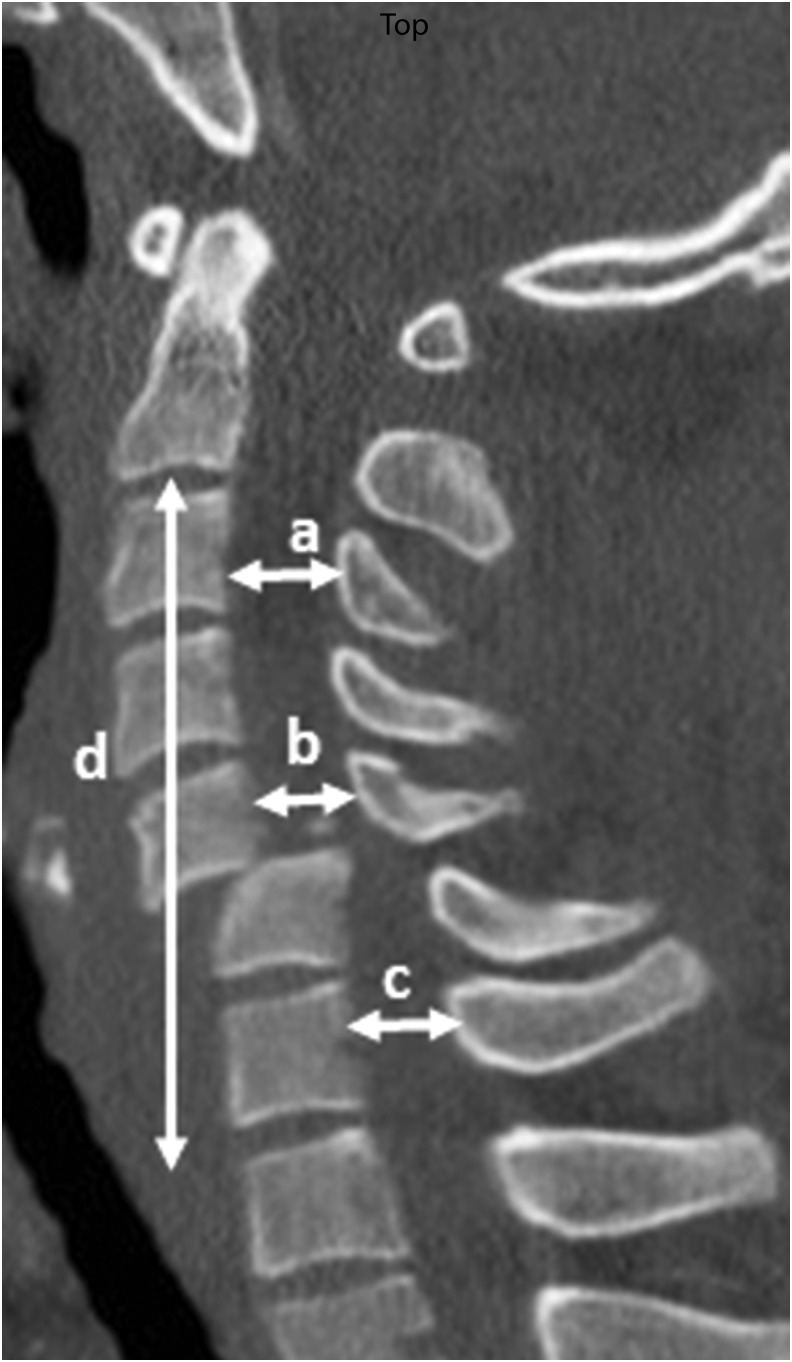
Midsagittal plane of CT scan from subaxial cervical spine indicating methodology of measurements of sagittal diameter at three segmental levels and the subaxial cervical spine height from C2-T1.
